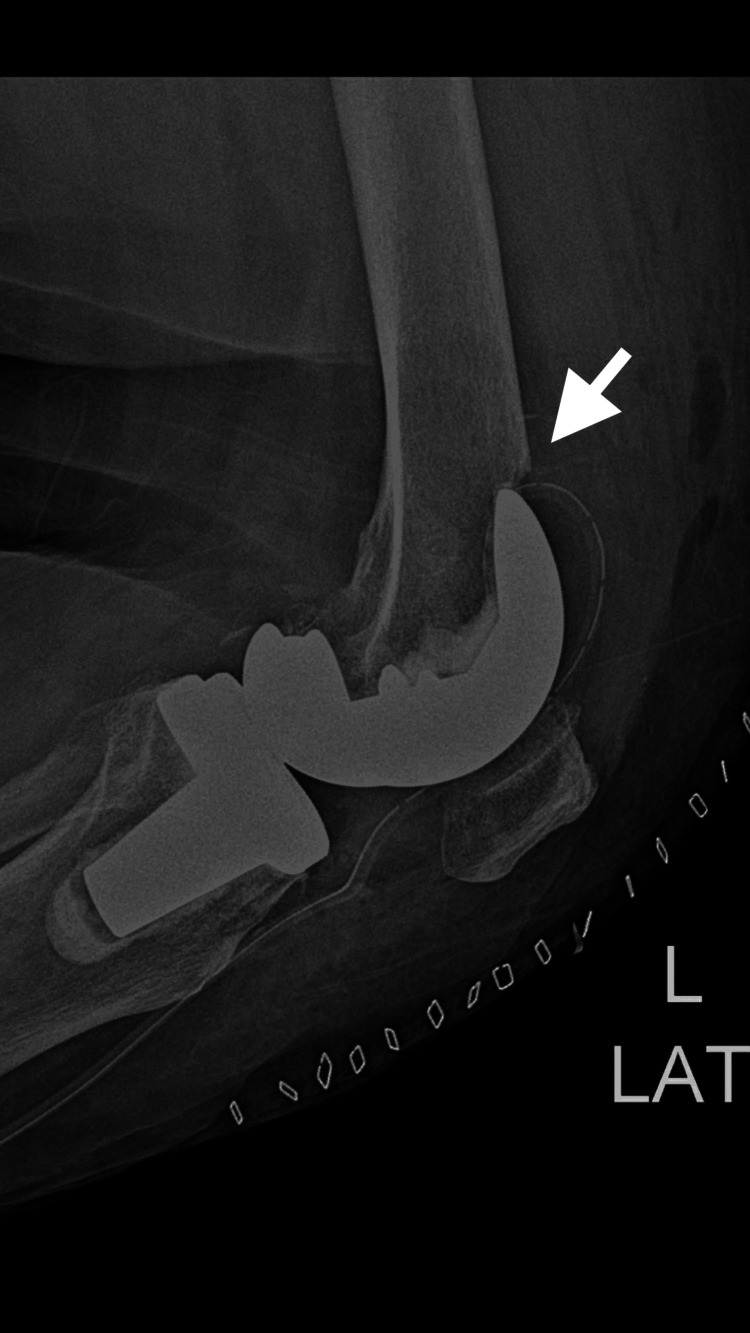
Immediate postoperative lateral radiograph of the left knee Immediate postoperative lateral radiograph of the left knee demonstrating anterior femoral cortical notching adjacent to the femoral component, which may represent a potential biomechanical stress riser.
